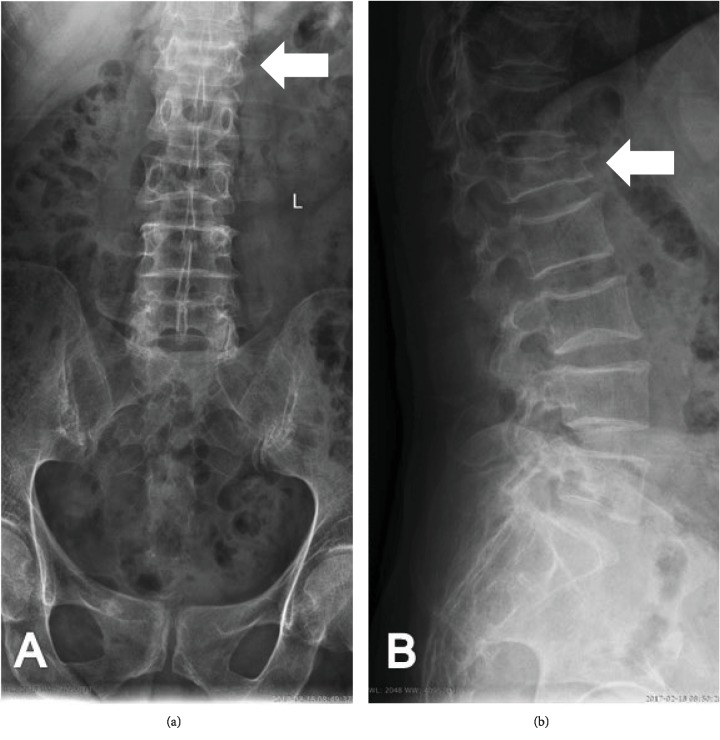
Radiographs showing the advanced osteoporosis with pathological fracture of the first lumbar vertebra (L1) in anteroposterior (a) and lateral (b) view. White arrows indicate the fracture site.
